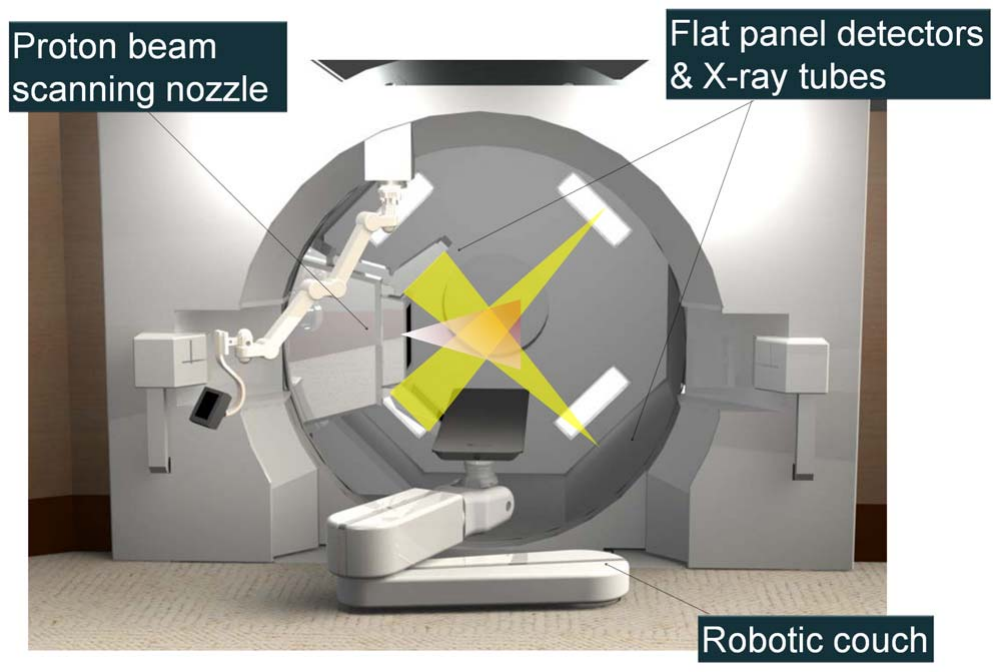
Design of a spot-scanning proton beam therapy-dedicated system with X-ray fluoroscopy. Two orthogonal sets of X-ray fluoroscopic generators and flat panels can be mounted in the gantry.
